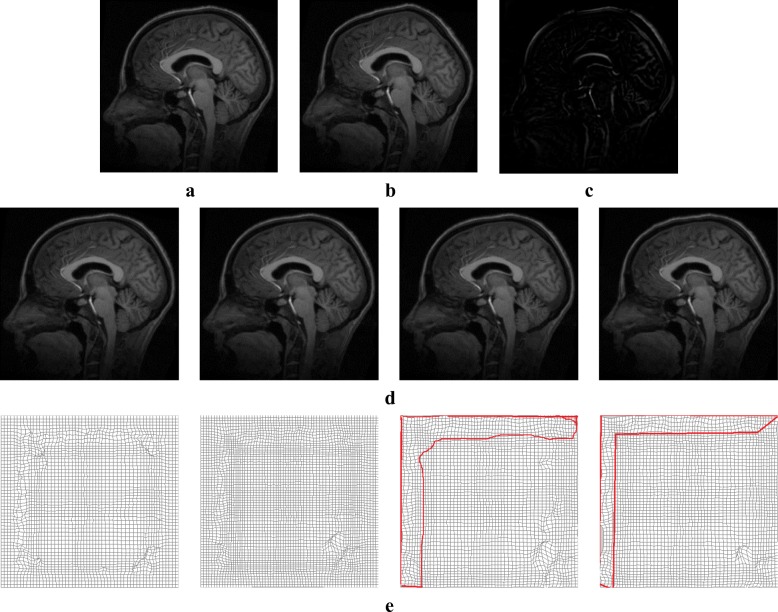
Registration result of MRI image: (a) reference image; (b) moving image; (c) initial difference between reference and moving images, (d) registration results obtained using our method, diffeomorphic demons, additive demons, and active demons, respectively, from left to right, and (e) final deformation field
